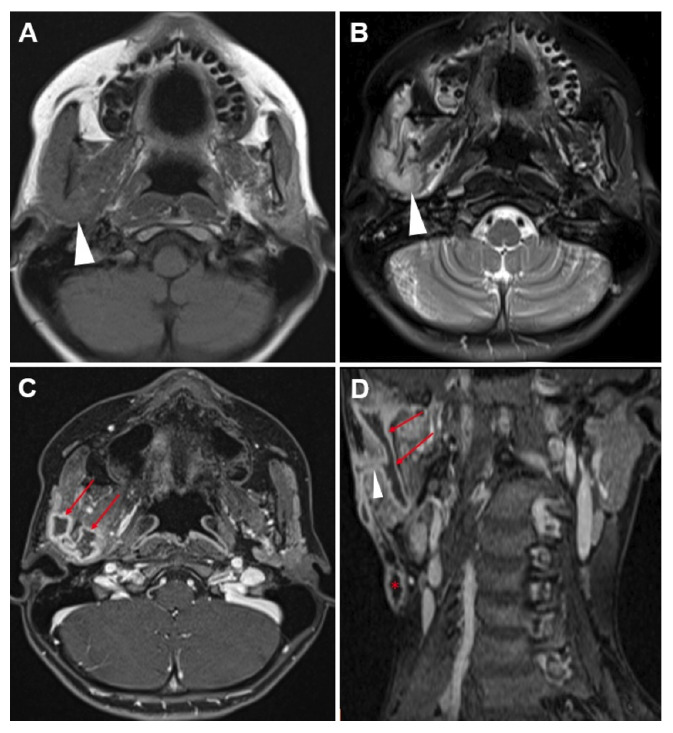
Axial MR images show a hypointense appearance on the T1-weighted image (A) and a hyperintense appearance (arrow) on the T2-weighted image (B) in the right masticatory space. Post-contrast T1-weighted axial (C) and coronal (D) sections demonstrate a peripherally contrast-enhancing abscess loculation (red arrows) and a fistula tract (arrowhead) extending to the skin. A central necrotic LAP formation (asterisks) is noted inferior to the lesion.
